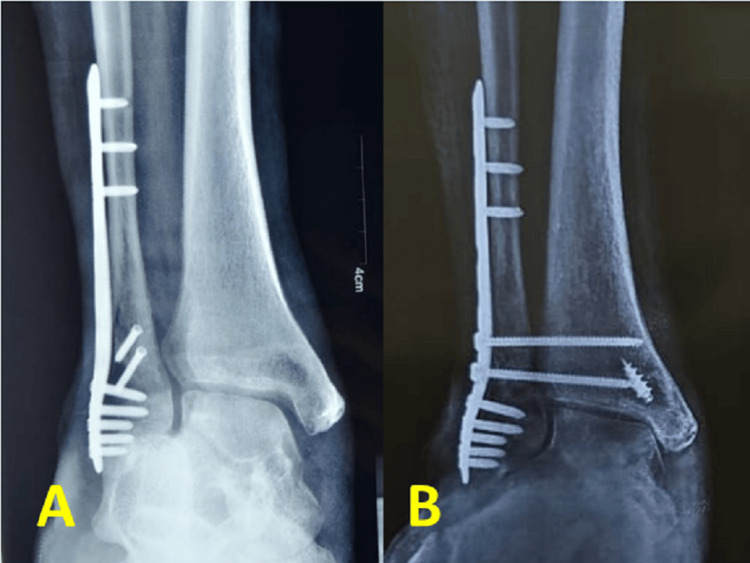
Preoperative (A) and postoperative X-rays (B) of a patient who underwent the quadrangular construct with syndesmotic stabilization and medial displacement calcaneum osteotomy.
